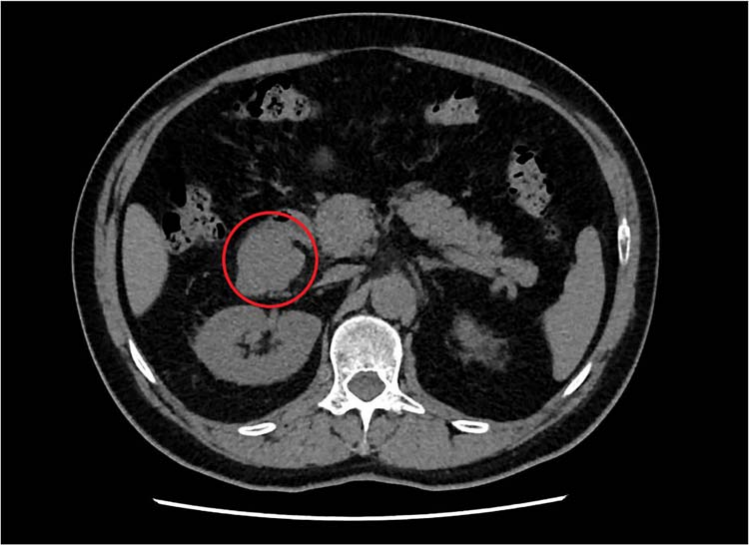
Transversal abdominal CT scan revealing a retroperitoneal polylobate neoformation in the right perirenal space.
